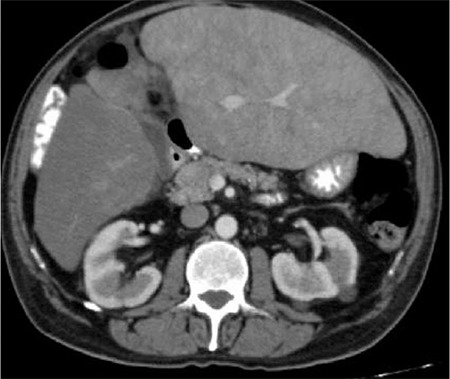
Abdominal CT angiography demonstrated occlusion of the left hepatic vein and enlargement in the left lobe of the liver.
